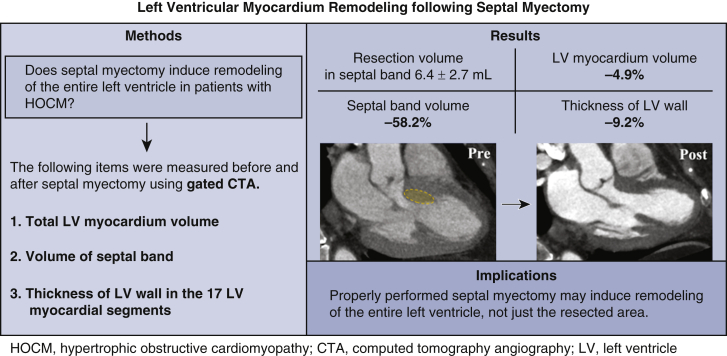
Gated cardiac computed tomography angiography (*CTA*) showed that whole left ventricle (*LV*) remodeling is induced after septal myectomy in patients with hypertrophic obstructive cardiomyopathy (*HOCM*).
